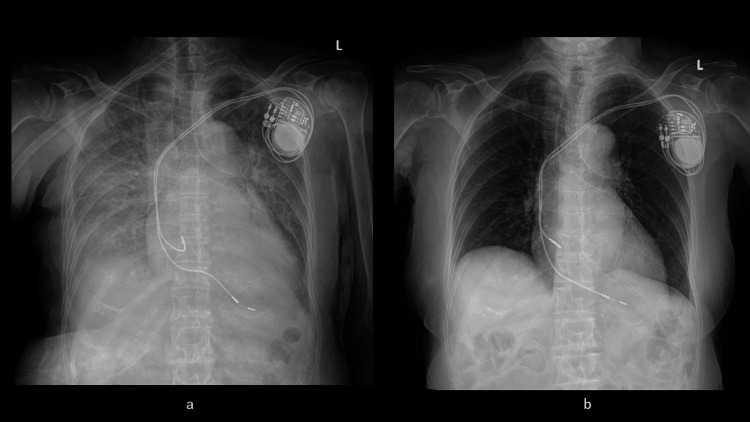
Chest X-ray films exhibiting pulmonary congestion and recovery a: Significant pulmonary congestion was observed due to substantial preload and mitral regurgitation caused by systolic anterior movement of the anterior leaflet. b: Pulmonary congestion improved with careful administration of diuretics and use of beta-blockers.
